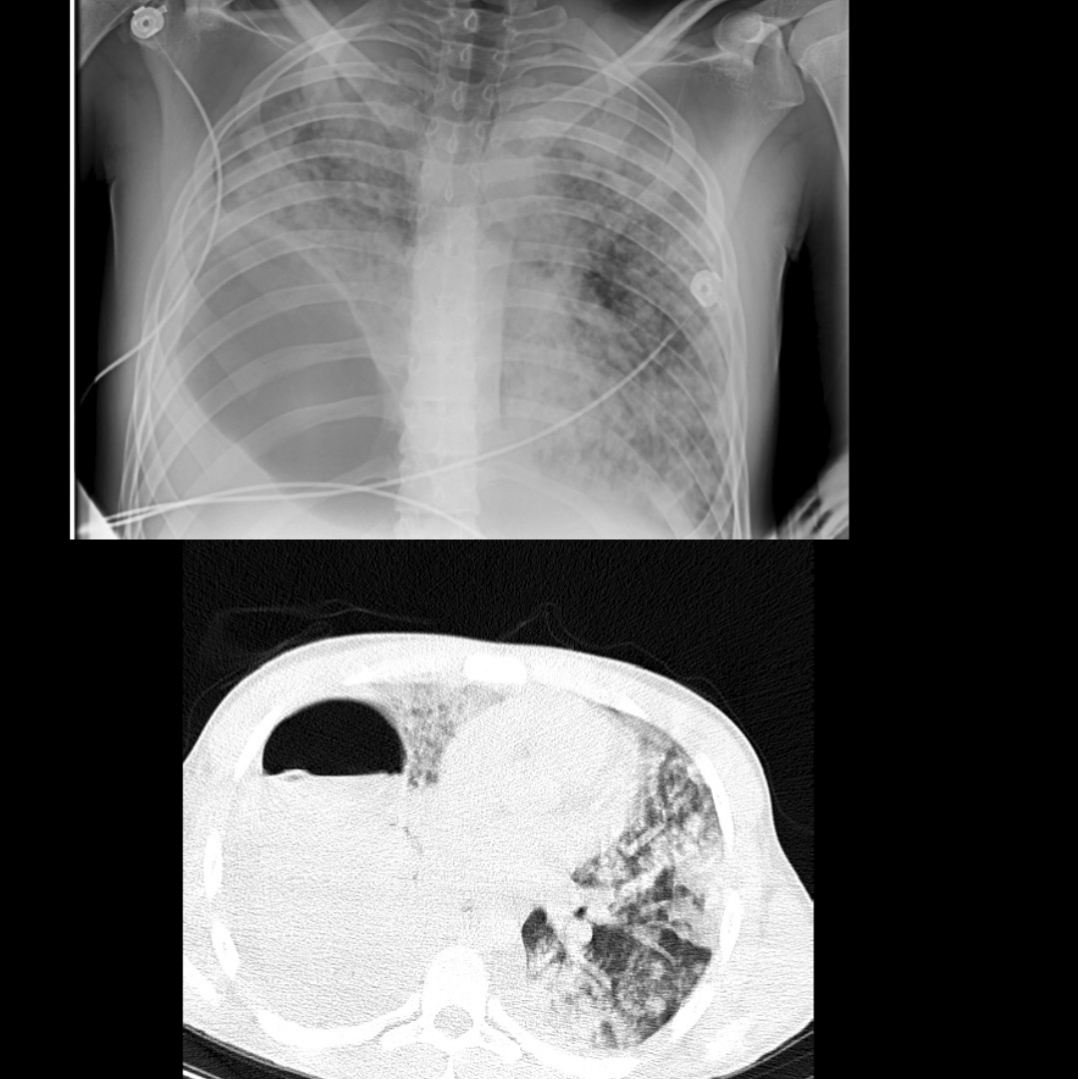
Chest X‐ray and Chest CT scan showing rupture of the cyst and diffuse ground glass opacities with centrilobular pattern in the field of both lungs suggesting ARDS.
